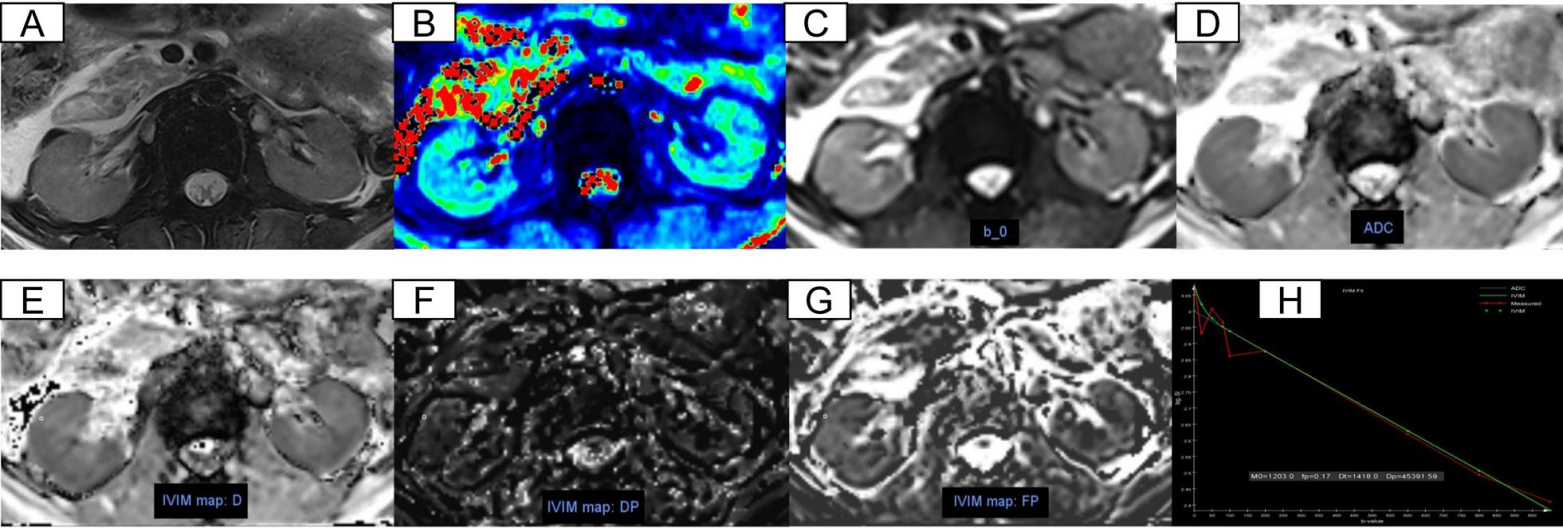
Respective MRI images of CKD stage 4–5 (13 years old, female). A–H Axial T2-weighted images, T2* mapping, b0 map, ADC, *D*_*t*_, *D*_*p*_, *f*_*p*_, IVIM fit, respectively
